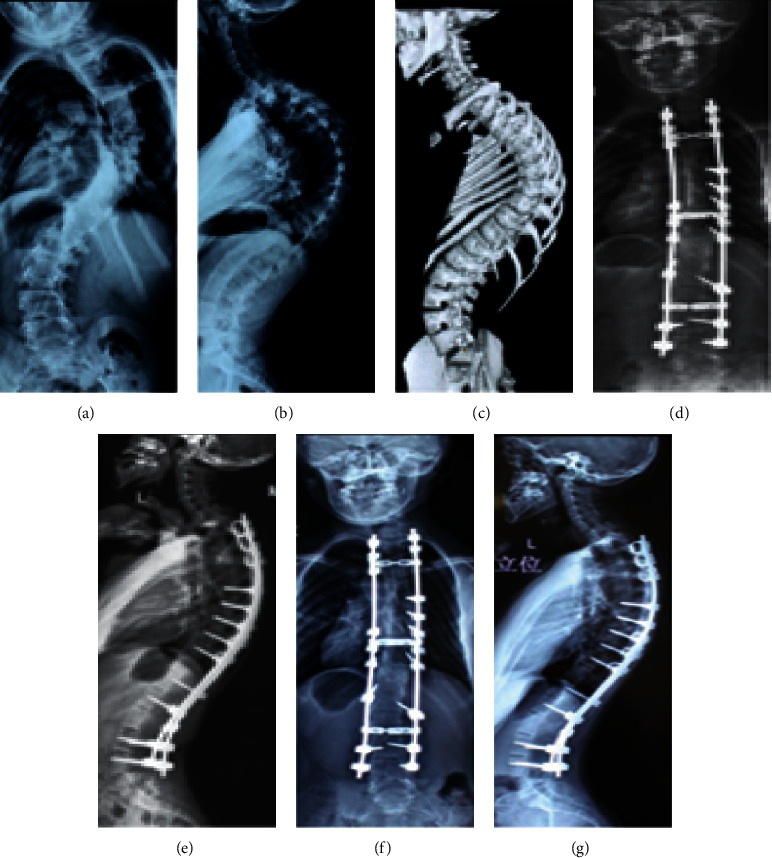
Radiographic films of a 40-year-old female patient with neglected scoliosis who underwent scoliosis curve correction and developed asymptomatic PJK after one year. (a) Preoperative anteroposterior (AP) X-ray views; (b) preoperative lateral X-ray view; and (c) a preoperative bone scan 3D model. (d, e) Immediate postoperative AP and lateral X-ray films and (f, g) AP and lateral X-ray films conducted after 1 year. The PJK angle after 1 year was 110 greater than immediate postoperative.
